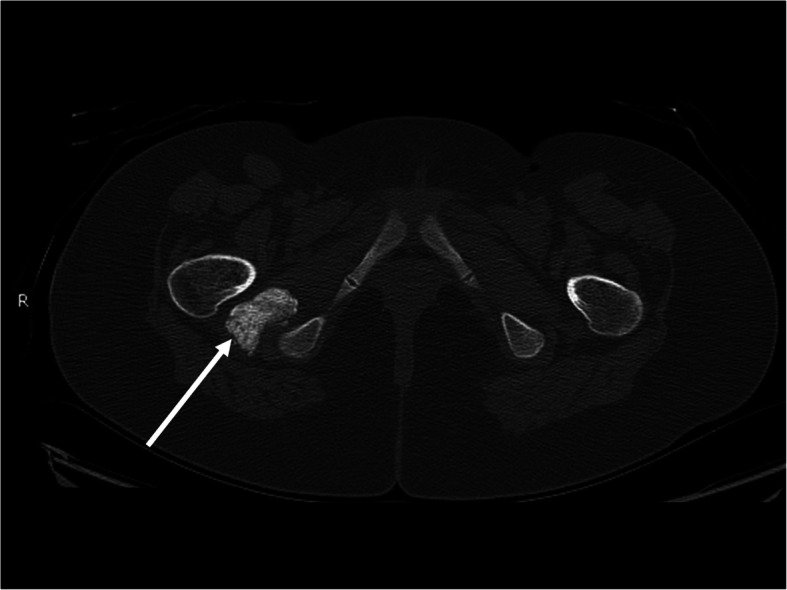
Axial CT image of the pelvis demonstrates a large area of bulky soft tissue calcification in the right ischiofemoral space (white arrow)
